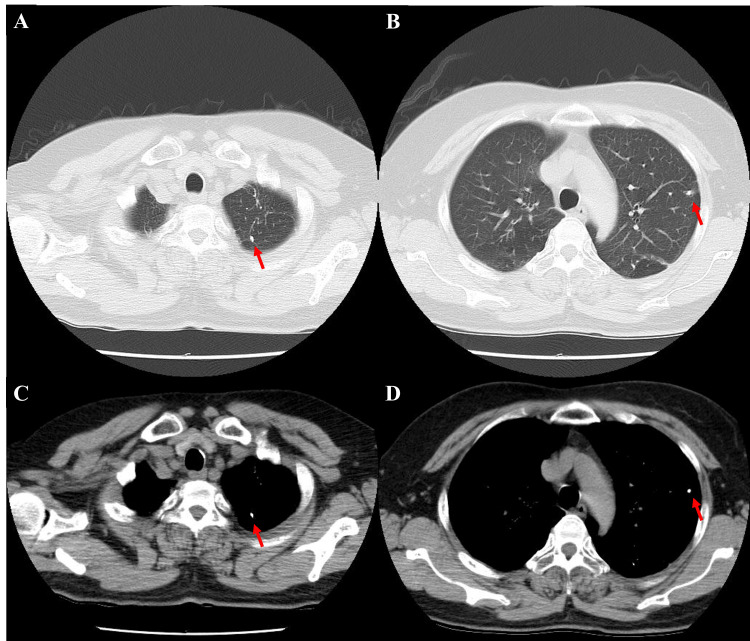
Chest computed tomography (CT) revealing nodules with calcification in the apical regions of the lungs (A, C) and the left upper lobe (B, D), consistent with old (inactive) tuberculosis.
